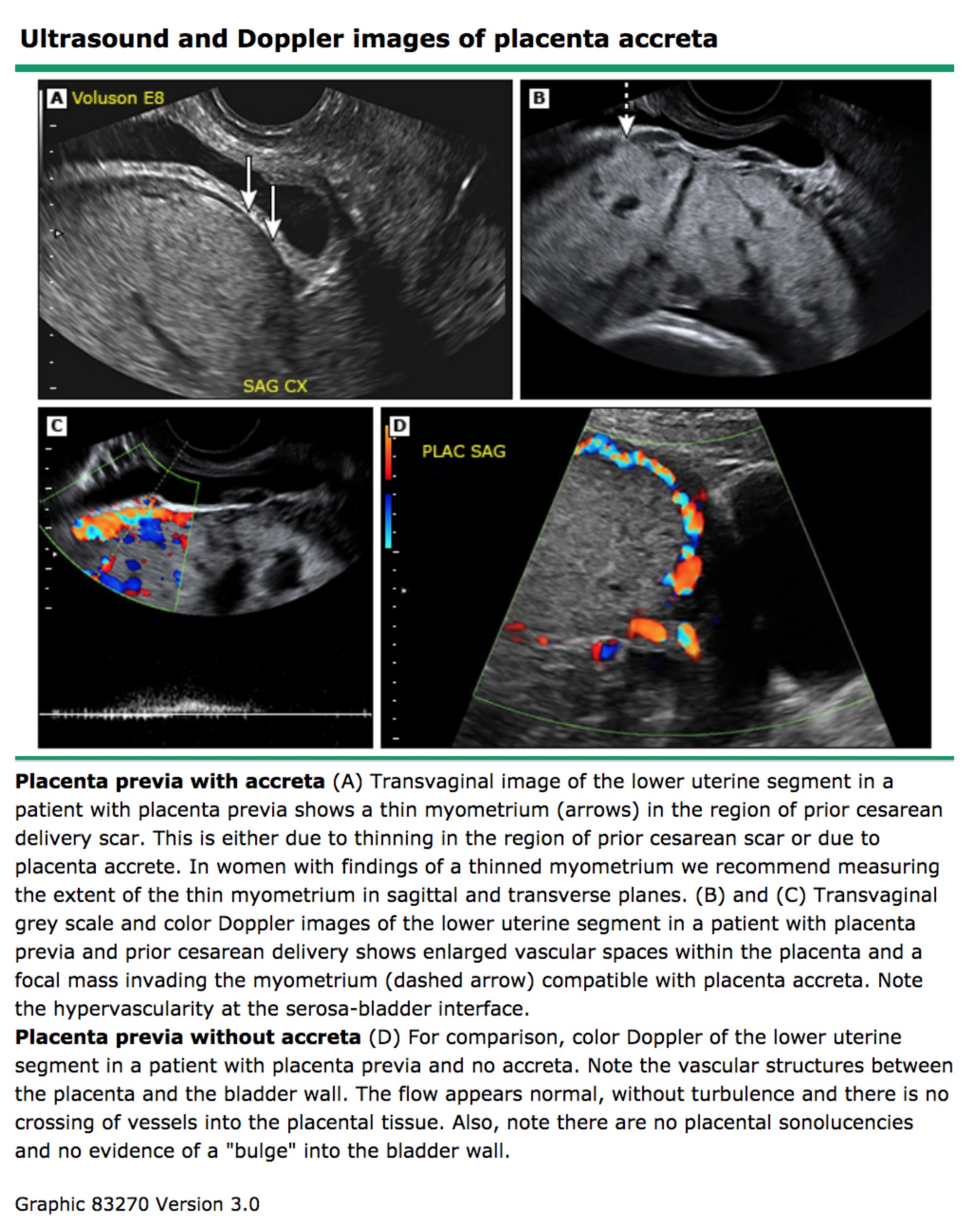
Ultrasound and Doppler images of placenta accreta. Reference [7] This figure was used from UpToDate with consent.
